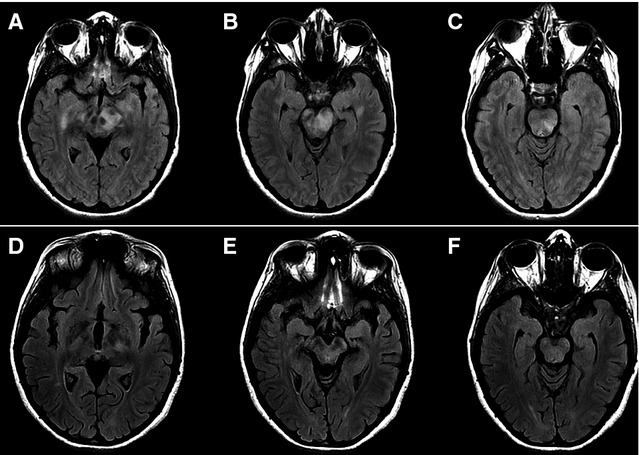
(A–C) MRI on axial Fast-FLAIR sequence shows demyelinating areas involving gyri rectus, mesencephalon, and pons. (D–F). Control MRI performed 15 months after initial symptoms shows improvement in demyelinating lesions and secondary cortical atrophy.
